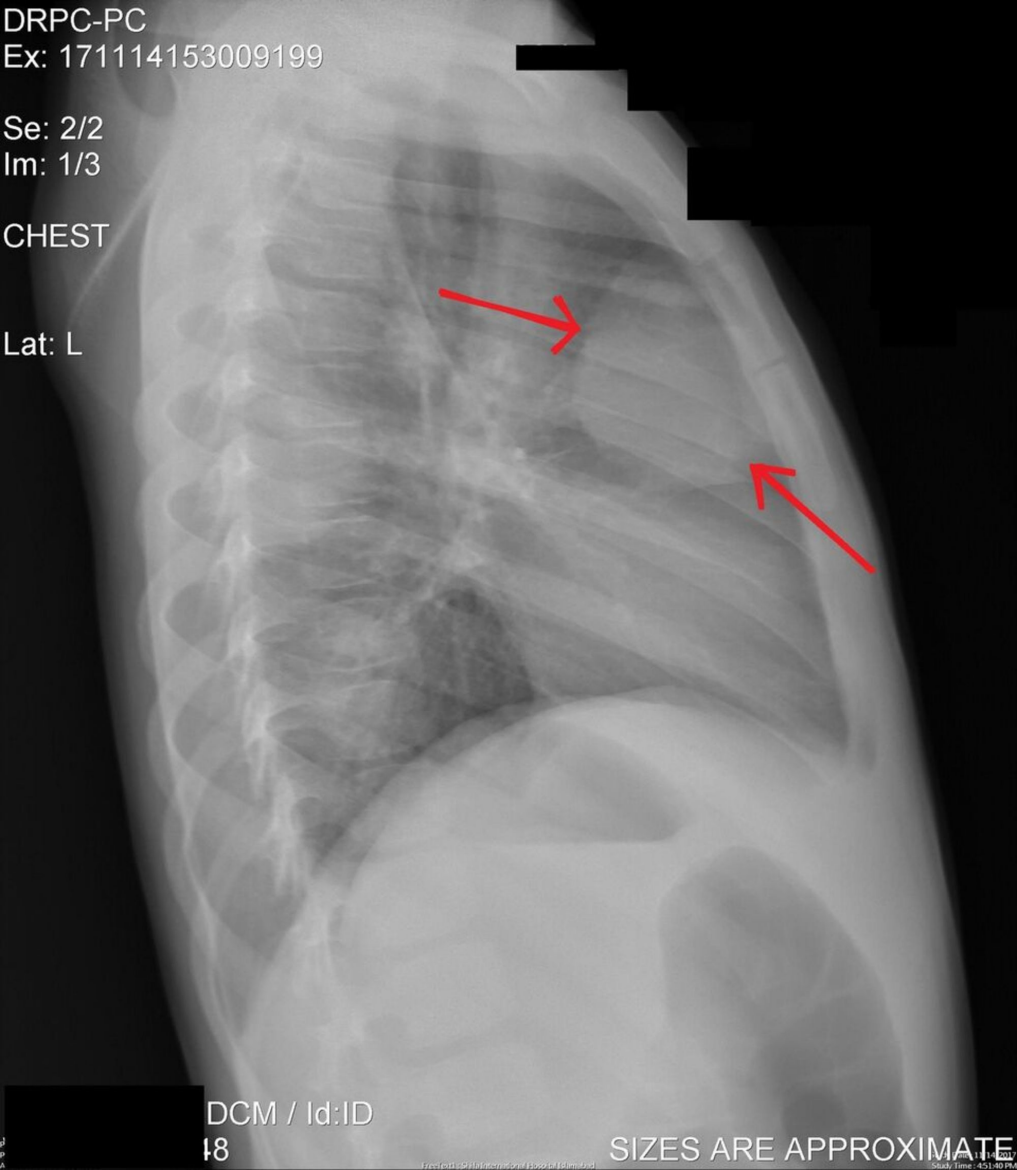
Chest X-ray (CXR) lateral view CXR lateral view showing anterior mediastinal mass.
